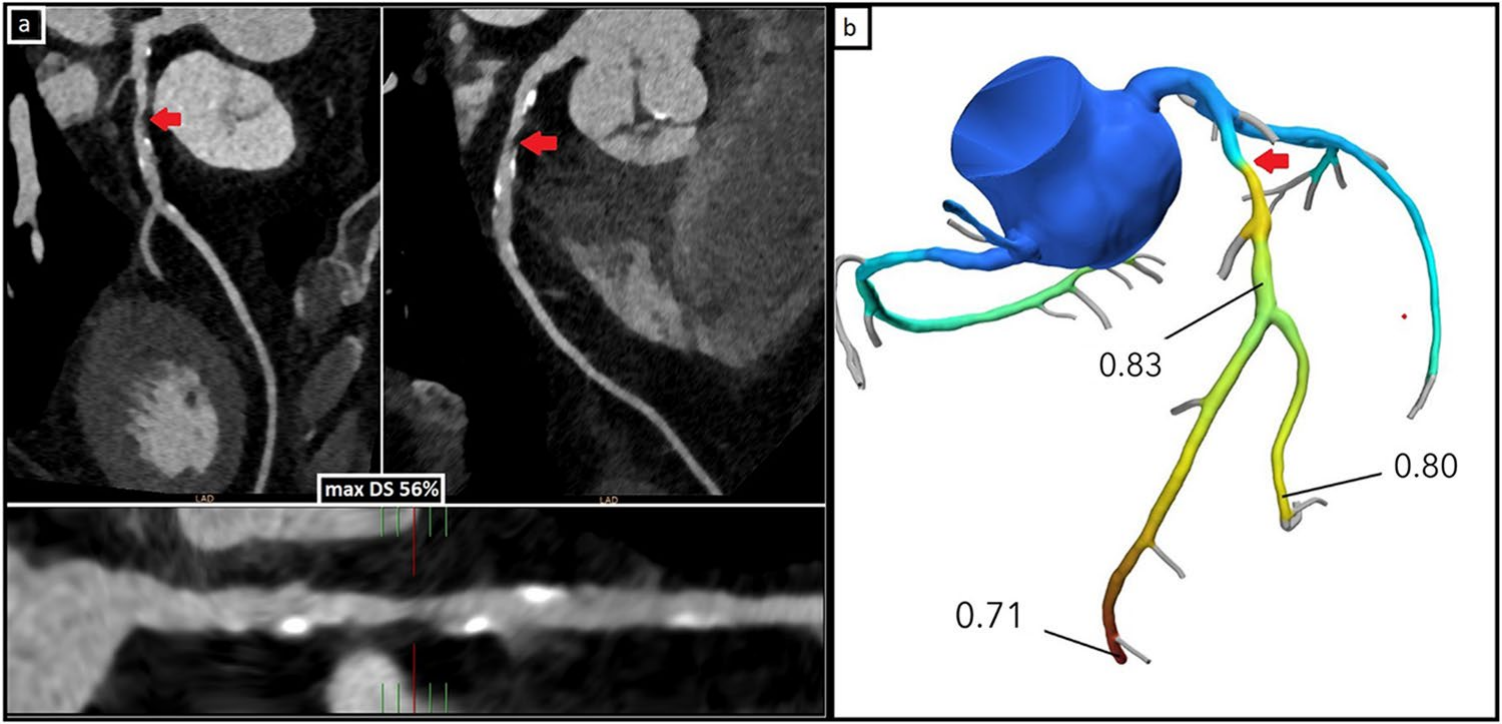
Example of significant stenosis on CCTA and negative FFR-CT result. a CCTA images of anatomically significant left anterior descending artery (LAD)-stenosis with maximal diameter reduction (max DS) 56%. b FFR-CT results for this CCTA. FFR-CT value after stenosis 0.83, which is negative for ischemia on FFR-CT. The red arrow points to the stenosis in all images. The patient was successfully treated with OMT only and was referred back to their general practitioner
